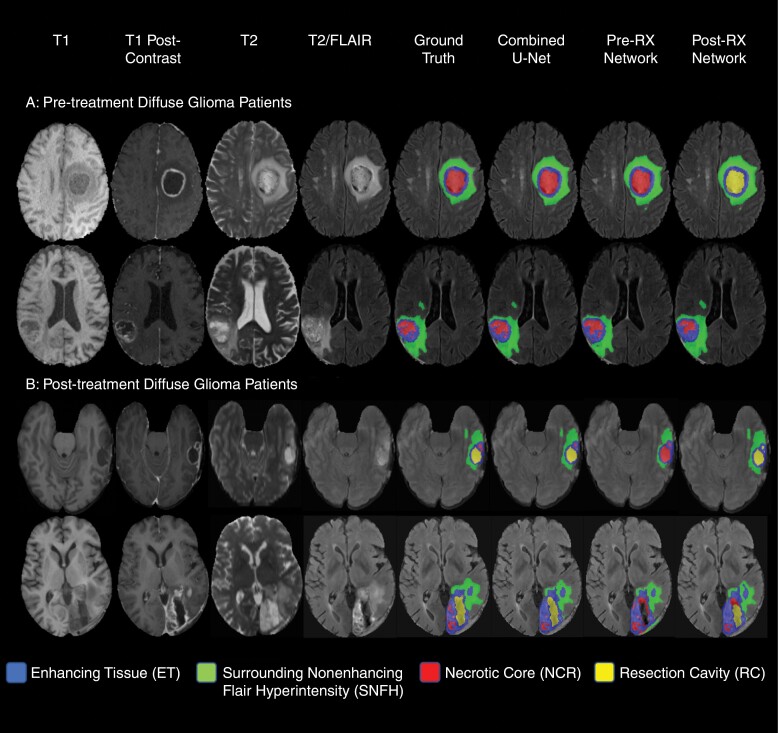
Example segmentation network predicted segmentations. Two example cases of pretreatment and posttreatment diffuse glioma MRIs with axial T2 images, T1 pre-contrast images (T1-pre), T1 post-contrast images (T1-post), FLAIR images, and example tumor tissue class segmentations overlaid on the FLAIR image (blue = enhancing tissue (ET), green = surrounding nonenhancing flair hyperintensity (SNFH), yellow = resection cavity (RC), and red = necrotic tumor core (NCR).
